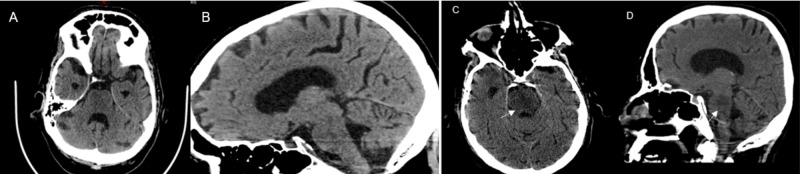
Initial and repeat non-contrast computed tomography (CT) scans of the brain (A) Axial and (B) sagittal views on a non-contrast CT head scan showing no abnormalities; (C) axial and (D) sagittal views on a non-contrast CT head scan three weeks later showing hypodensity (arrow) in the central pons, suggesting central pontine myelinolysis (CPM)
